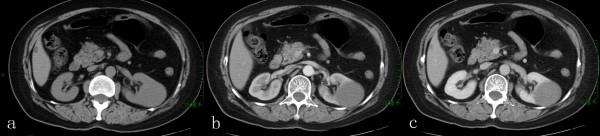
Contrast enhanced abdominal CT scan. It revealed a tumor on the middle portion of the kidney (a), and demonstrated the tumor was slightly enhanced on the corticomedullary phase (b) and nephrographic phase (c).
